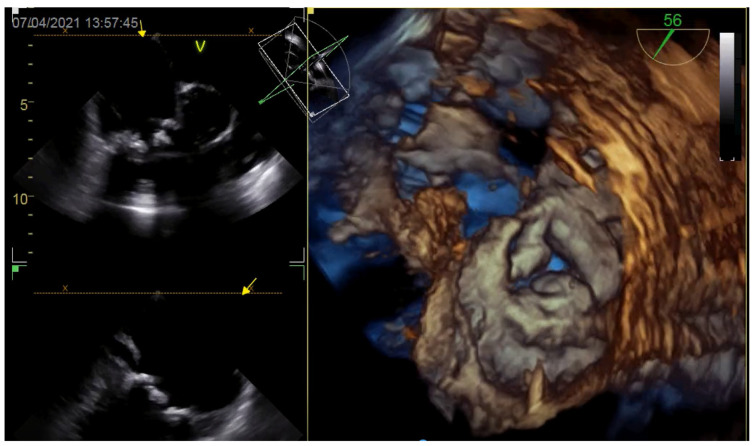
Three-dimensional transesophageal echocardiography showing the severely stenosed and regurgitant tricuspid bioprosthesis.
